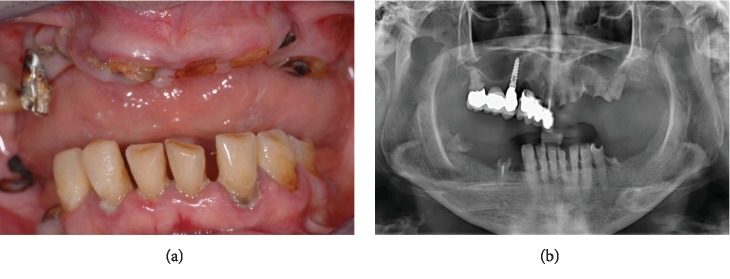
(a) Baseline clinical view of the severely compromised residual dentition; (b) baseline orthopantomograph.
